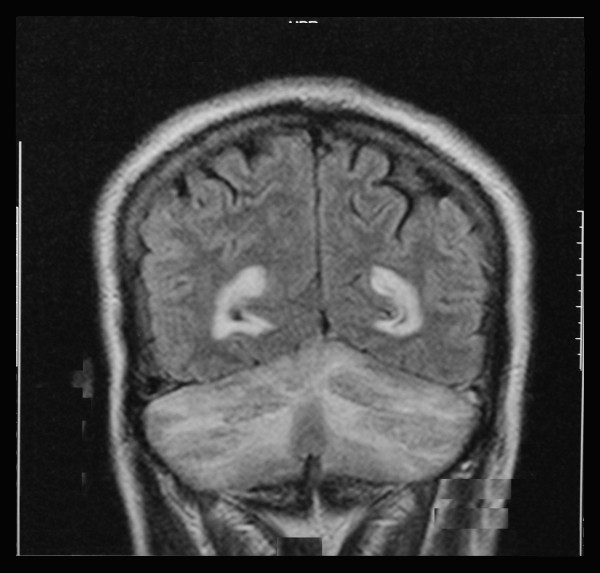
Coronal magnetic resonance imaging showing hyperintense areas in his cerebellar white matter in a T2-weighted sequence.
